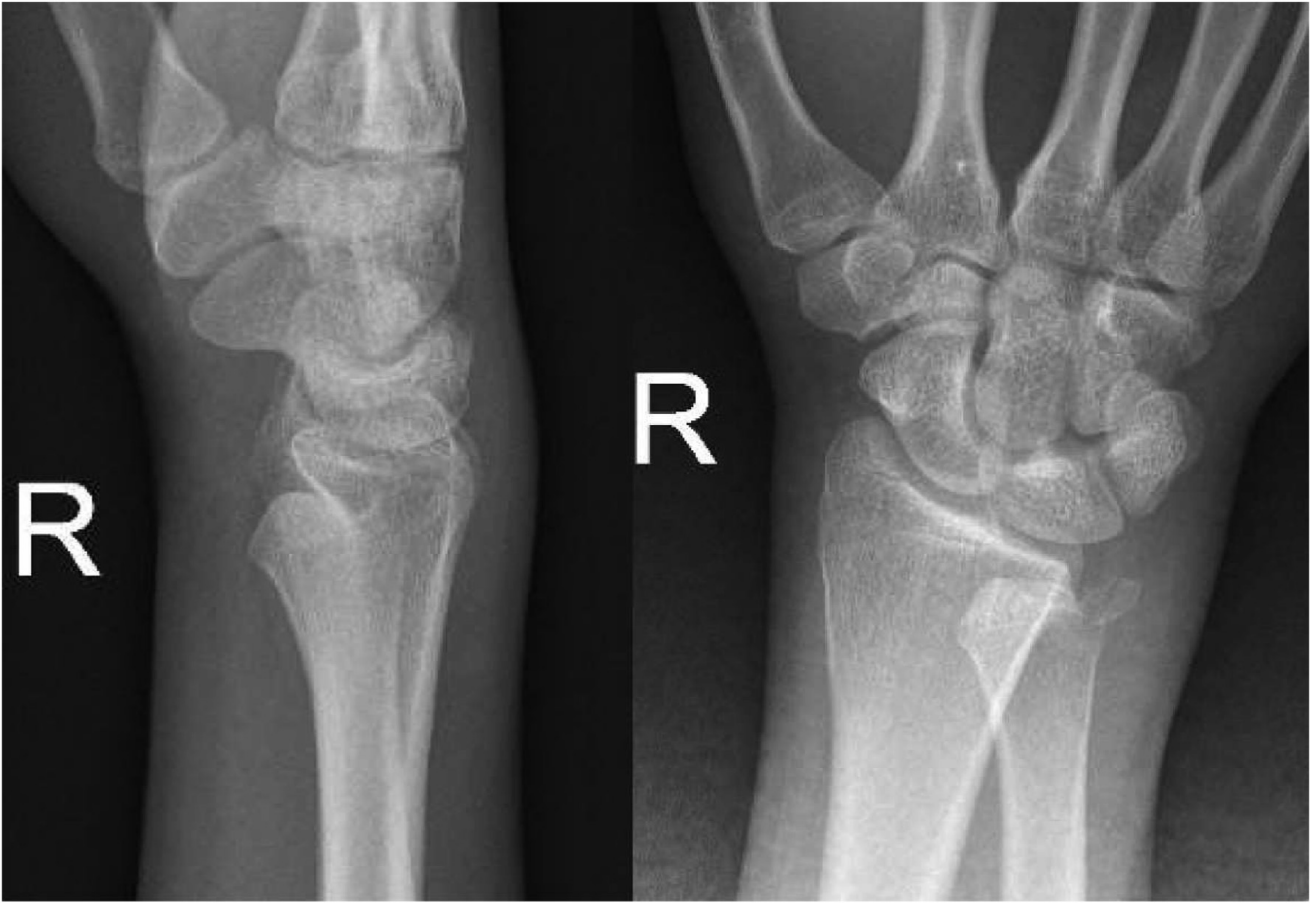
Radiographs of the wrist at initial presentation.
